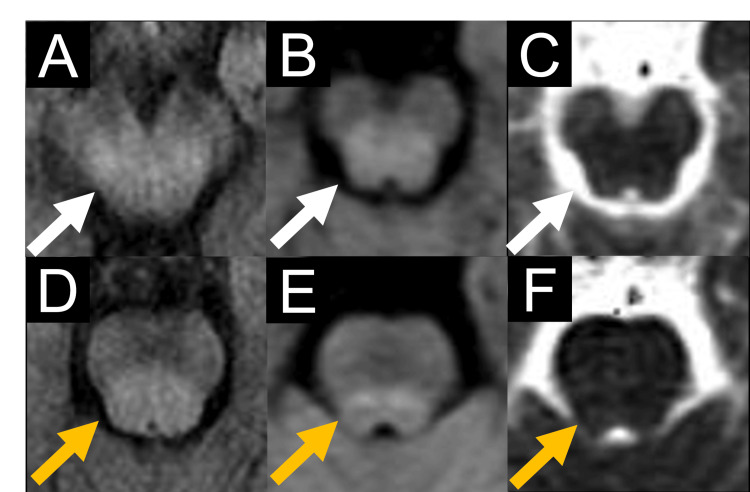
MRI findings on day 3 of admission MRI showed remained hyperintensity in the midbrain tegmentum, sparing the cerebral crus, on FLAIR imaging (A), along with hyperintensity on DWI (B) and low values on the ADC map (C) (white arrows). Furthermore, MRI revealed hyperintensity in the pontine tegmentum on FLAIR (D), with corresponding hyperintensity on DWI (E) and low values on the ADC map (F) (orange arrows). ADC: Apparent diffusion coefficient; DWI: Diffusion-weighted imaging; FLAIR: Fluid-attenuated inversion recovery
